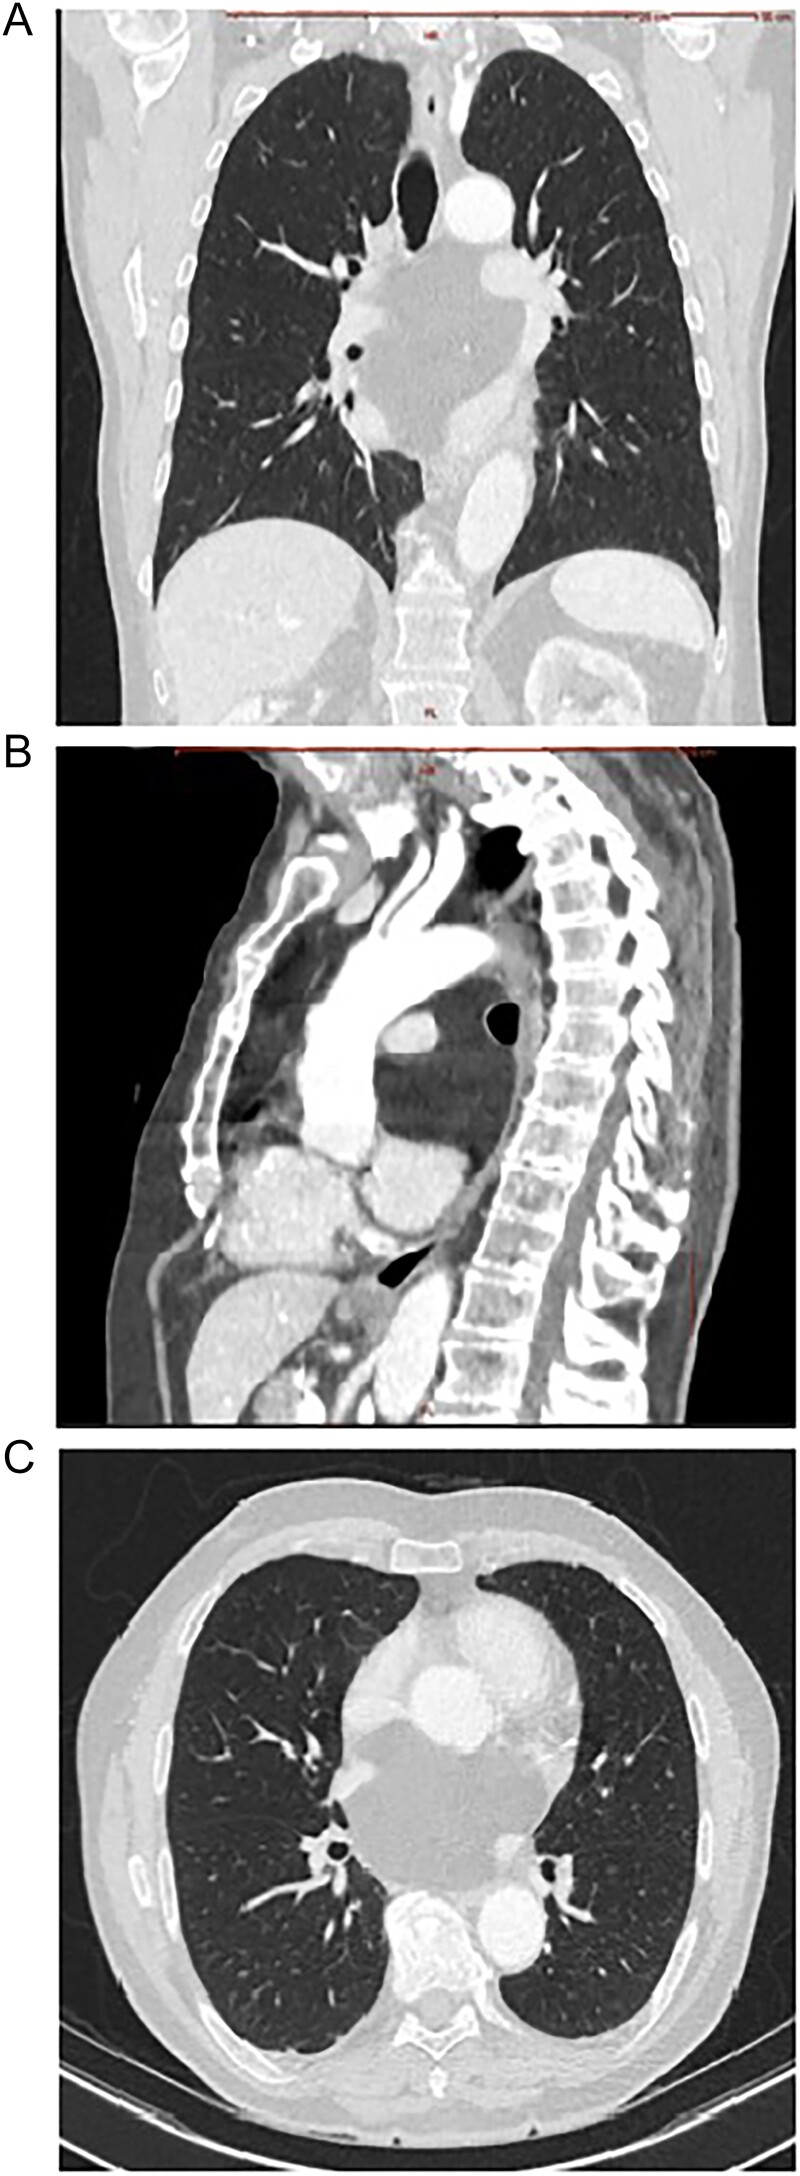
Contrast enhanced CT scan of mediastinum demonstrating the well-defined focal fat-attenuated homogenous lesion. (A) Coronal view, (B) sagittal view, (C)axial view.
